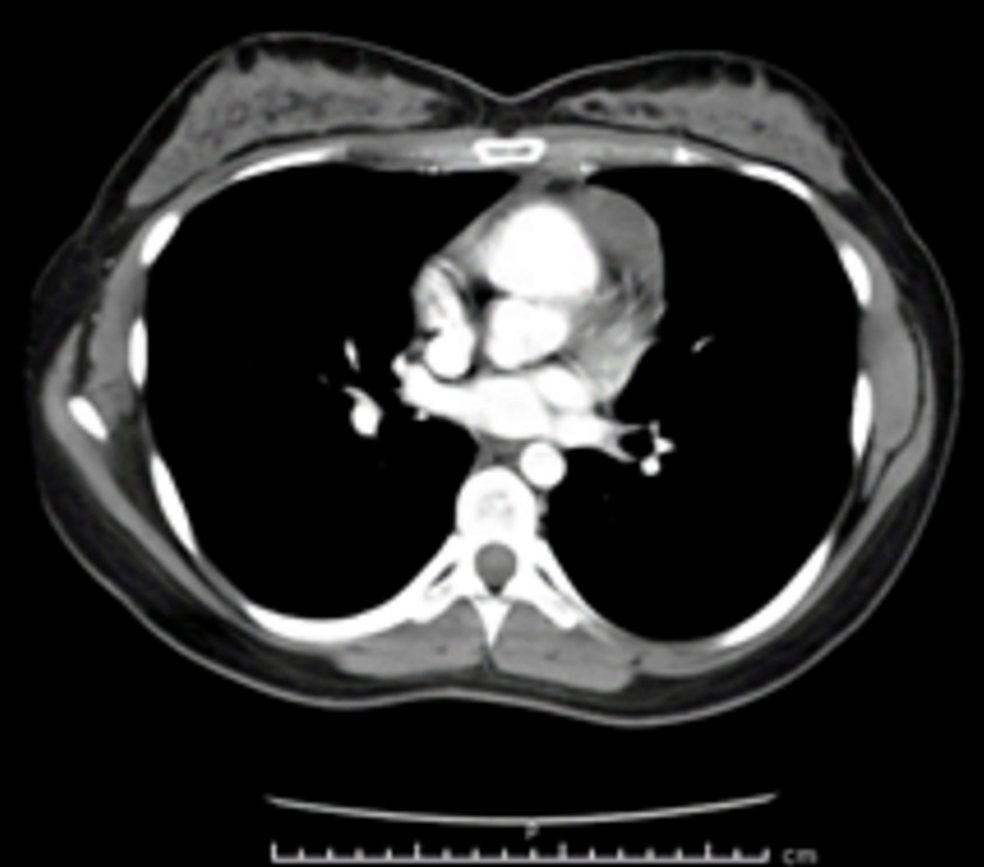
Chest Computed Tomography showing extensive prominent bulky heterogenous lymph nodes in the anterior and middle mediastinum (measures 8 cm in AP dimension and 7 cm transversely), including involvement of the pre-tracheal and perivascular spaces. As a result of the extensive involvement, there is significant narrowing of the left brachiocephalic vein as it traverses the mediastinum. There is also narrowing of the superior vena cava. No clear evidence of hilar adenopathy.
